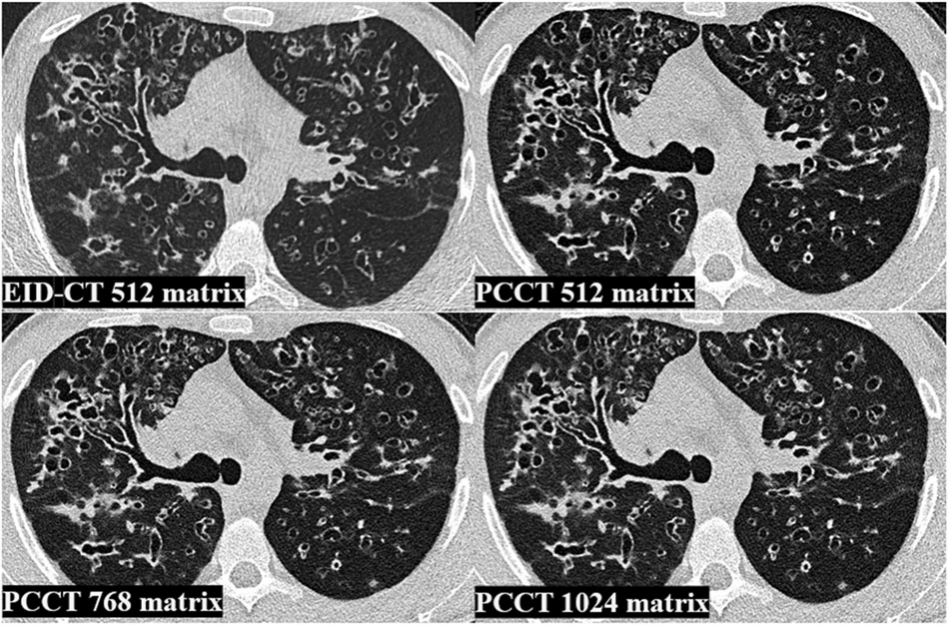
Examples of different reconstructions of chest CT of lower lobes with bronchiectasis and bronchial wall thickening. EID-CT, Energy-integrating detector CT; EID-CT 512; EID-CT 512 × 512 matrix; PCCT, Photon-counting CT; PCCT 512, PCCT 512 × 512 matrix; PCCT 768, PCCT 768 × 768 matrix; PCCT 1,024, PCCT 1,024 × 1,024 matrix
